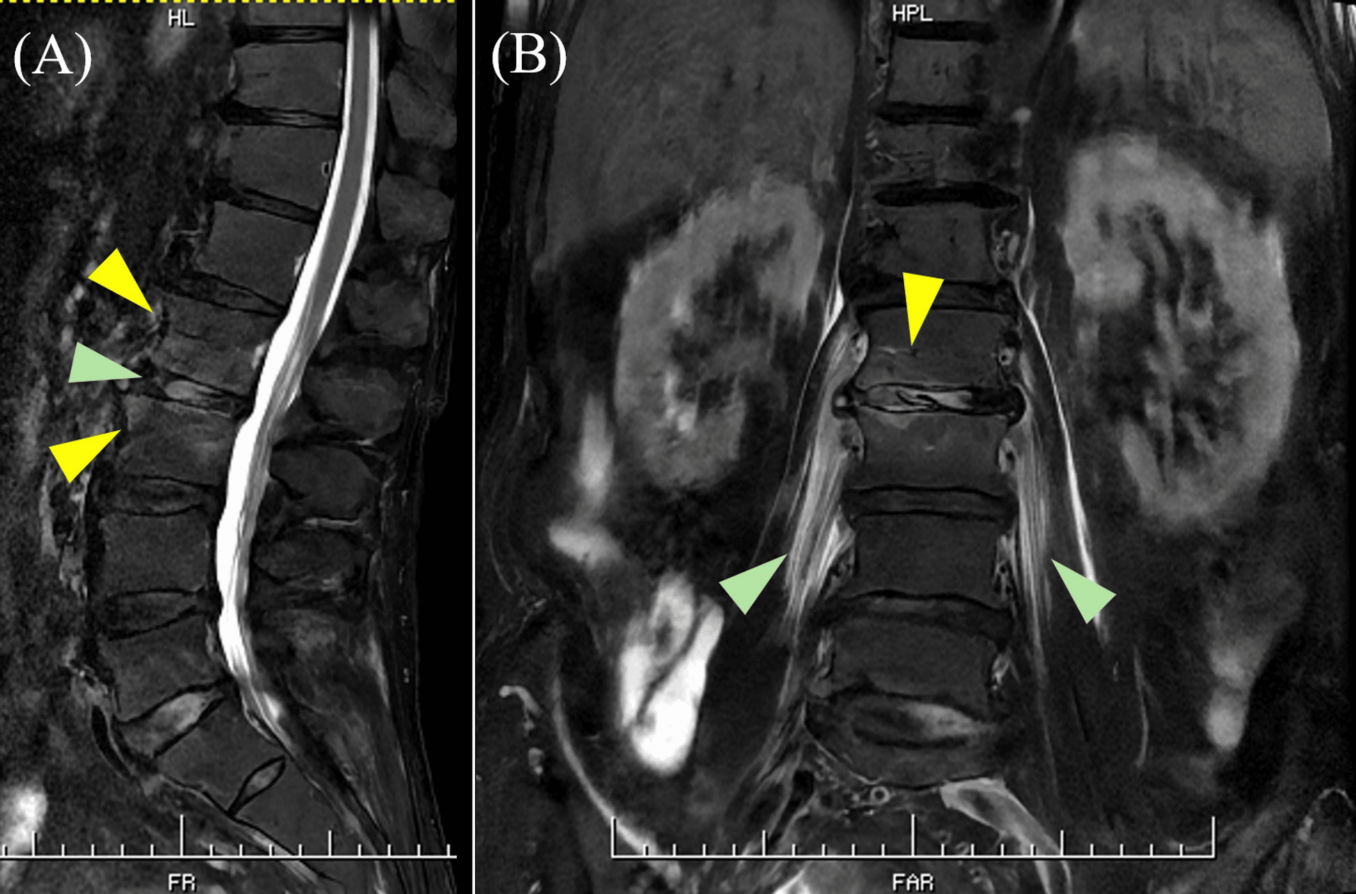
T2-weighted lumbar magnetic resonance imaging (MRI) on day 60. (A) Sagittal section of lumbar MRI on T2-weighted imaging showing hyperintensities at the L2 and L3 vertebral bodies (yellow arrowheads) and the L2/3 disc (green arrowhead). (B) Coronal section of lumbar MRI on T2-weighted imaging showing hyperintensities in the bilateral iliopsoas muscles (green arrowheads), the L2/3 disc (yellow arrowhead), and the L2 and L3 vertebral bodies (yellow arrowhead).
